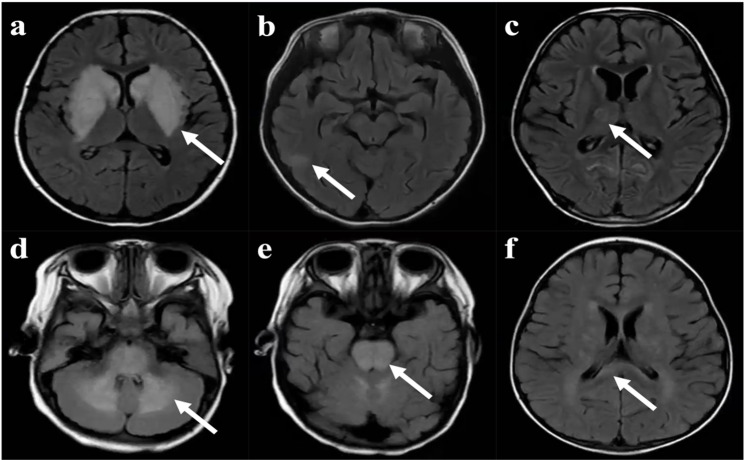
Brain MRI of patients with *Mycoplasma pneumoniae* encephalitis showing lesions in different locations. (a) Basal ganglia, T2 FLAIR, axial scan; (b) Cerebral cortex and adjacent white matter, T2 FLAIR, axial scan; (c) Thalamus, T2 FLAIR, axial scan; (d) Cerebellar hemisphere, T2 FLAIR, axial scan; (e) Brainstem, T2 FLAIR, axial scan; (f) Corpus callosum, T2 FLAIR, axial scan. *FLAIR* Fluid-Attenuated Inversion Recovery, *MRI* magnetic resonance imaging
